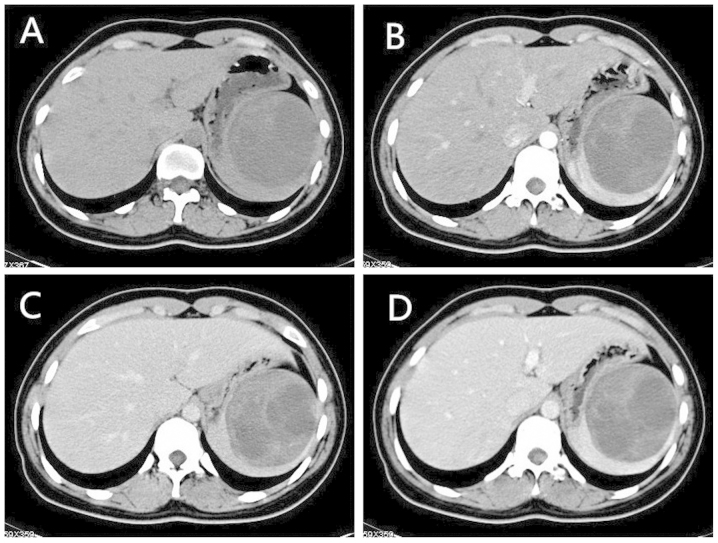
Computed tomography (CT) images. (A) Pre-contrast CT revealing a large, solitary, well-defined mass in the spleen, with variable areas of necrosis and cystic degeneration. Contrast-enhanced CT revealing the progressively-enhanced cystic wall, internal septa and solid portion during the (B) hepatic arterial, (C) portal venous and (D) hepatic parenchymal phases. The areas of necrosis and cystic degeneration were non-enhancing.
